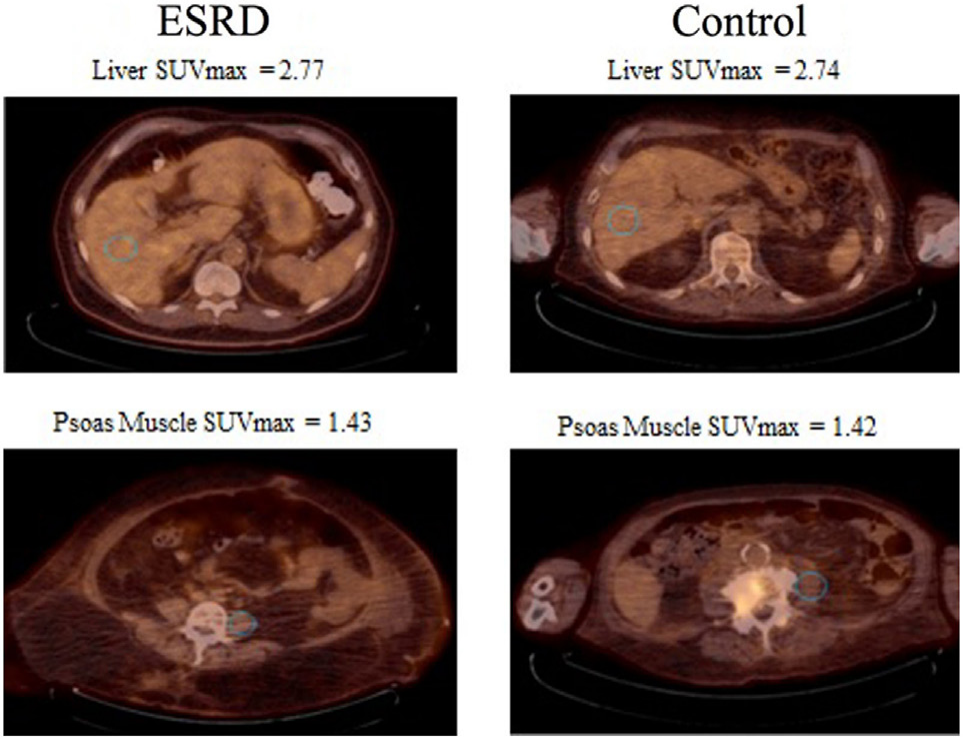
30 mm circular regions of interest (blue) were placed. The first in the right hepatic lobe and second in the left psoas muscle. In addition, the figure shows the mean standard uptake values (SUVs) between the controls and the renal failure patients.
